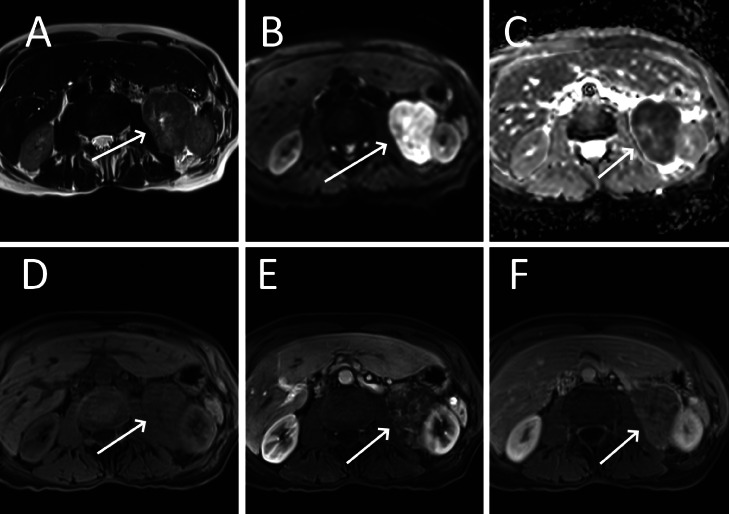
MRI of a para-renal tumor (arrows) that is heterogeneously hyperintense in T2-weighted imaging (A), restricts diffusion on high b-value (B), is hypointense in ADC imaging (C), is isointense in T1-weighted imaging (D) but enhances arterial (E) and venous phases (F).
